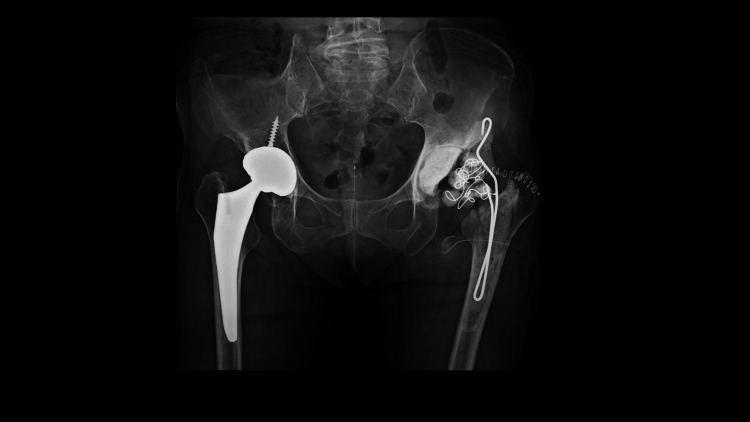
Hip radiograph from the first surgery All implants in the left hip joint were removed, and an antibiotic-loaded cement spacer was inserted into the acetabular and femoral canal.
